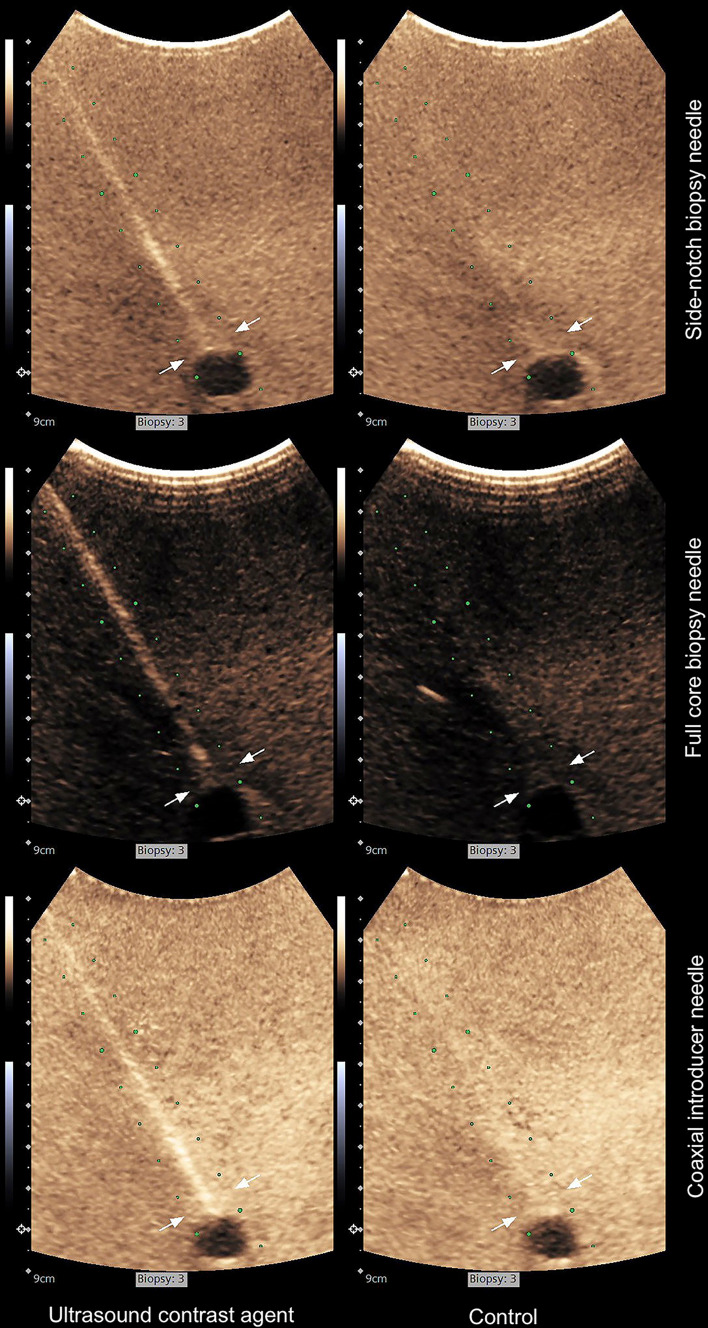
The first pairs of punctures (primed with ultrasound contrast agent versus controls) from the first of the three punctures in the first of the ten sets for the three needle types studied in the contrast-specific imaging-mode, as presented for the assessors. From the image frames with the needles in their end positions (end positions marked with white arrows), the median ones were selected for this set of images
